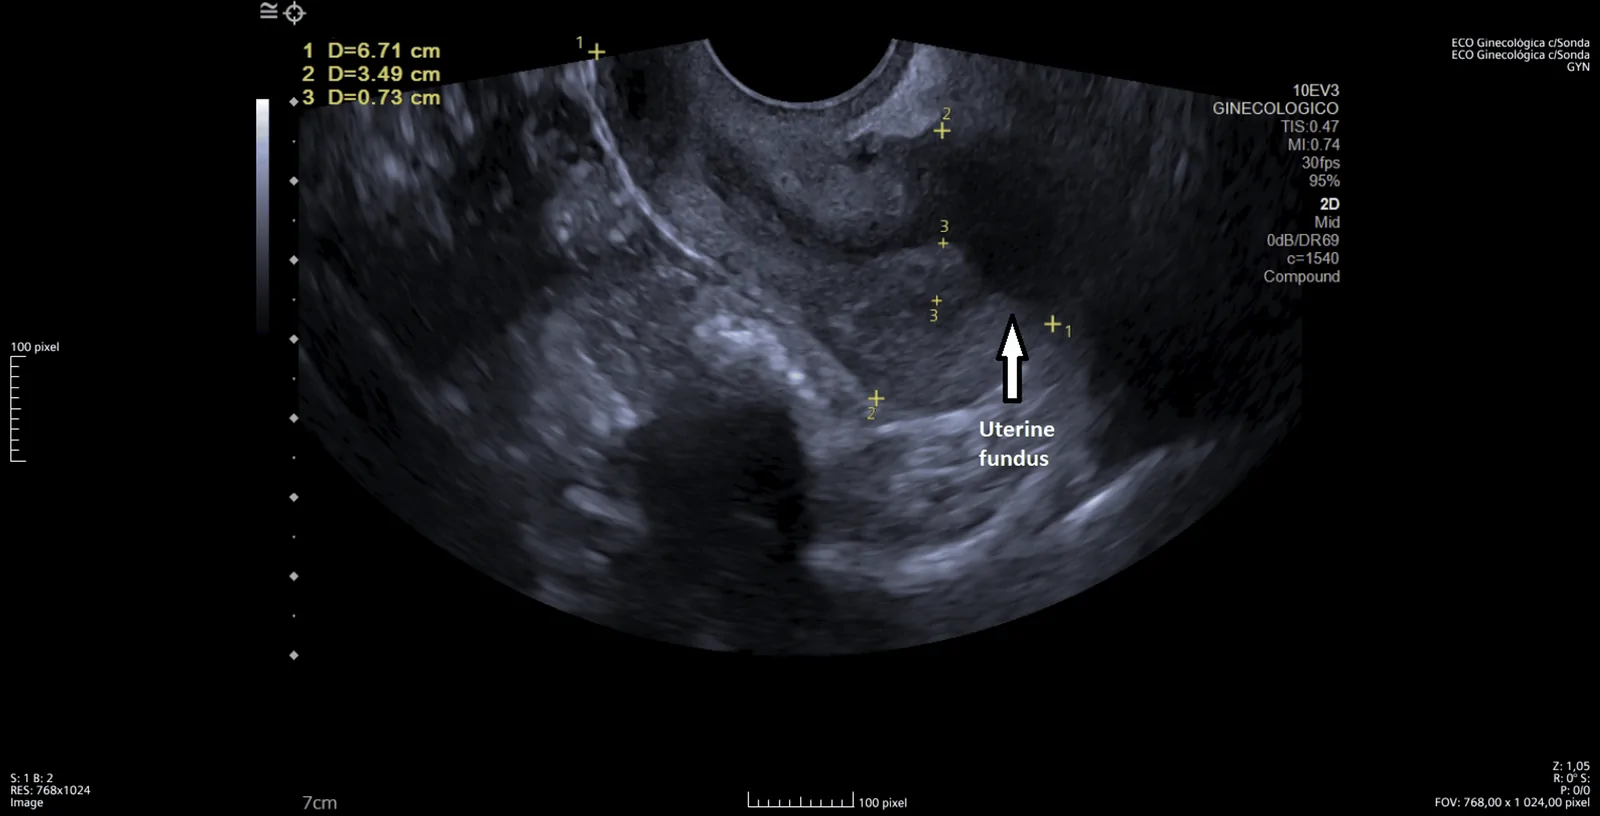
Initial TVUS demonstrating a retroflexed uterus with apparently normal uterine longitudinal dimensions (67 mm) prior to LNG-IUS insertion. The white arrow indicates the uterine fundus. TVUS: Transvaginal ultrasound; LNG-IUS: Levonorgestrel-releasing intrauterine system
